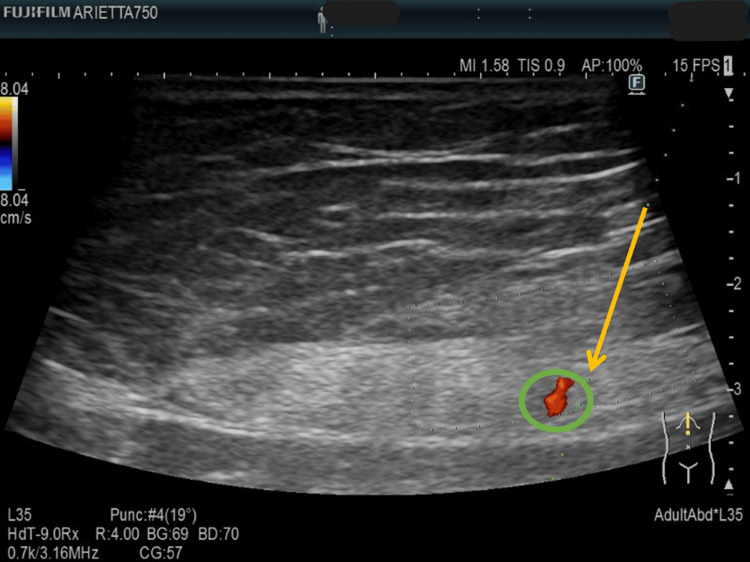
Ultrasound-guided hydrodissection targeting the anterior branch of the intercostal nerve at the point of maximal tenderness The needle insertion path (yellow arrow) was directed toward the perforating artery (green circle) at its pulsation point, targeting the anterior branch of the intercostal nerve penetration site located directly beneath the point of maximal tenderness with a positive Carnett’s sign.
